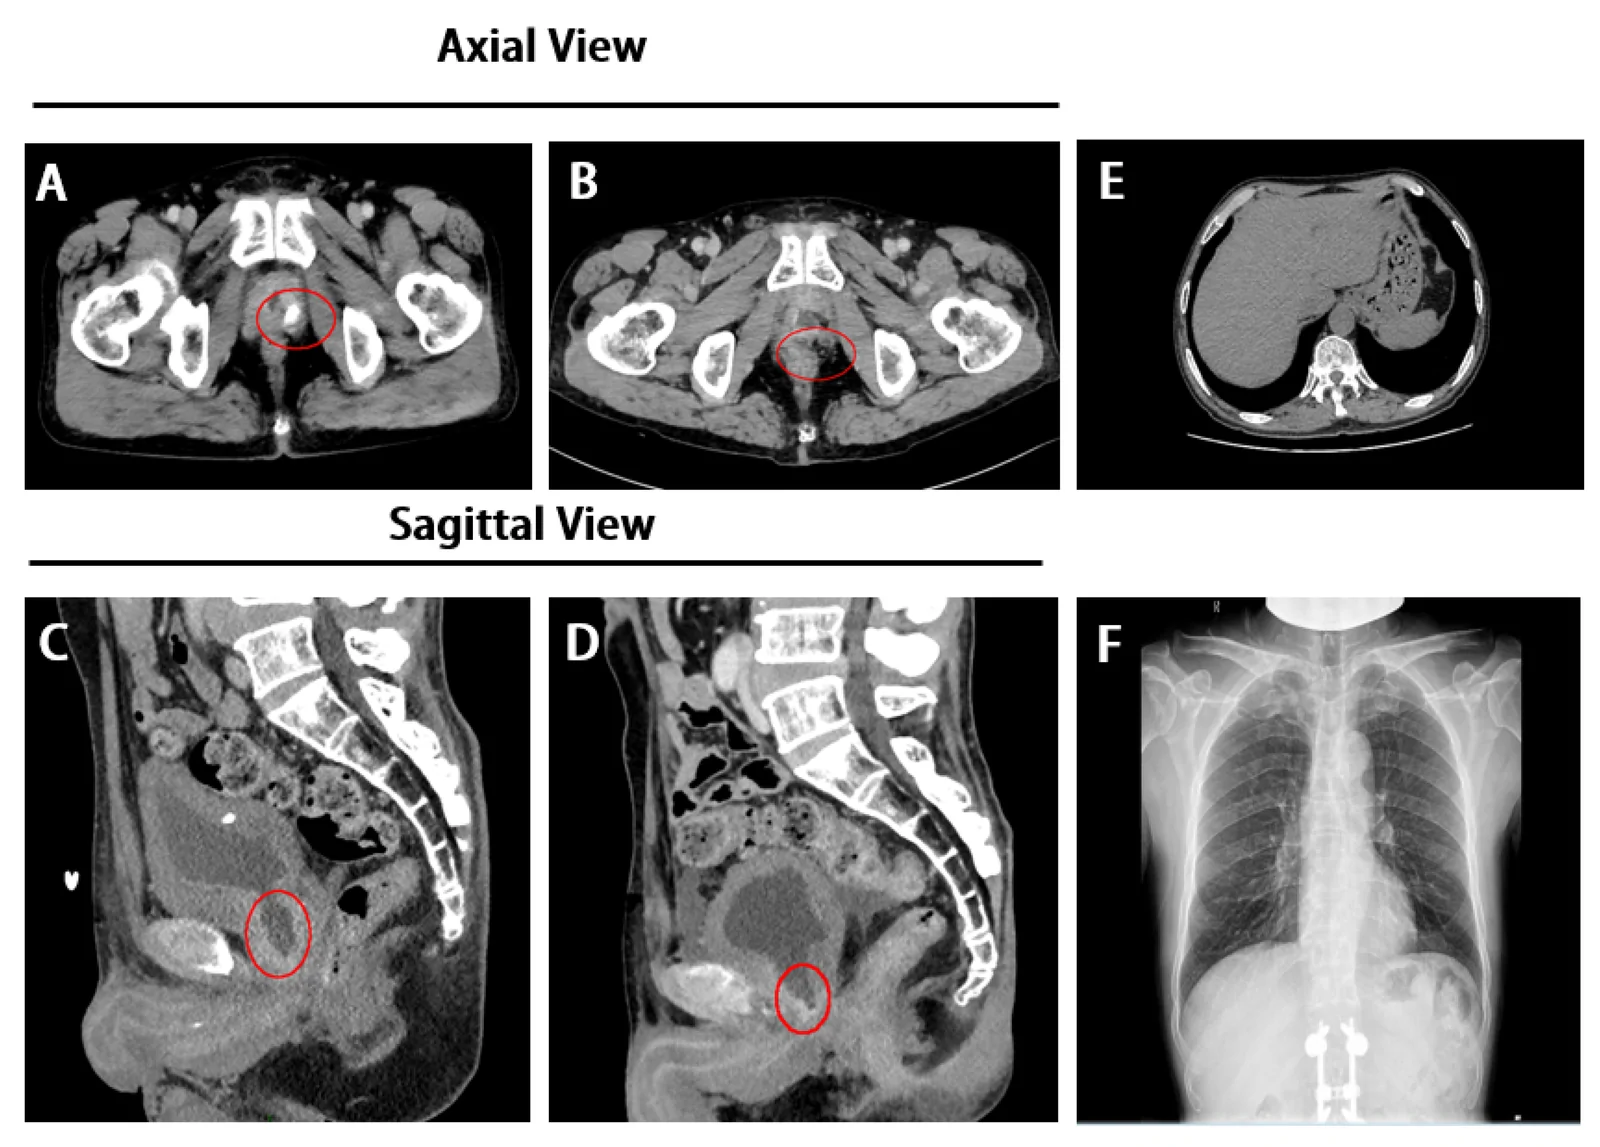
Preoperative contrast-enhanced pelvic computed tomography (CT) images, including axial (A) and sagittal (C) views, revealed an irregular soft-tissue lesion in the prostate/bladder neck region (red circles). Postoperative follow-up pelvic CT images, including axial (B) and sagittal (D) views, showed no definite residual nodular lesion in the corresponding region (red circles). Preoperative upper abdominal CT (E) revealed no focal hepatic lesion or other evidence of upper abdominal metastasis. Preoperative chest radiography (F) showed no focal pulmonary lesion suspicious for metastasis.
